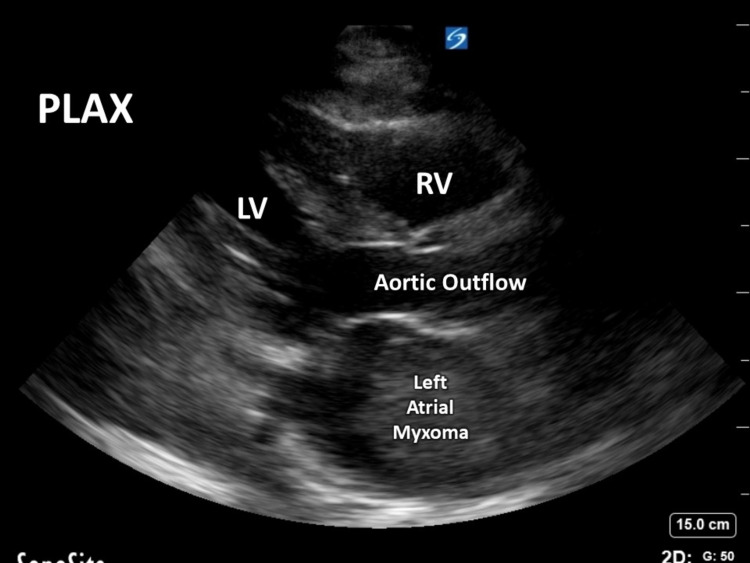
Transthoracic echocardiogram (TTE) left atrial myxoma parasternal long-axis view PLAX - parasternal long-axis view, RV - right ventricle, LV - left ventricle
